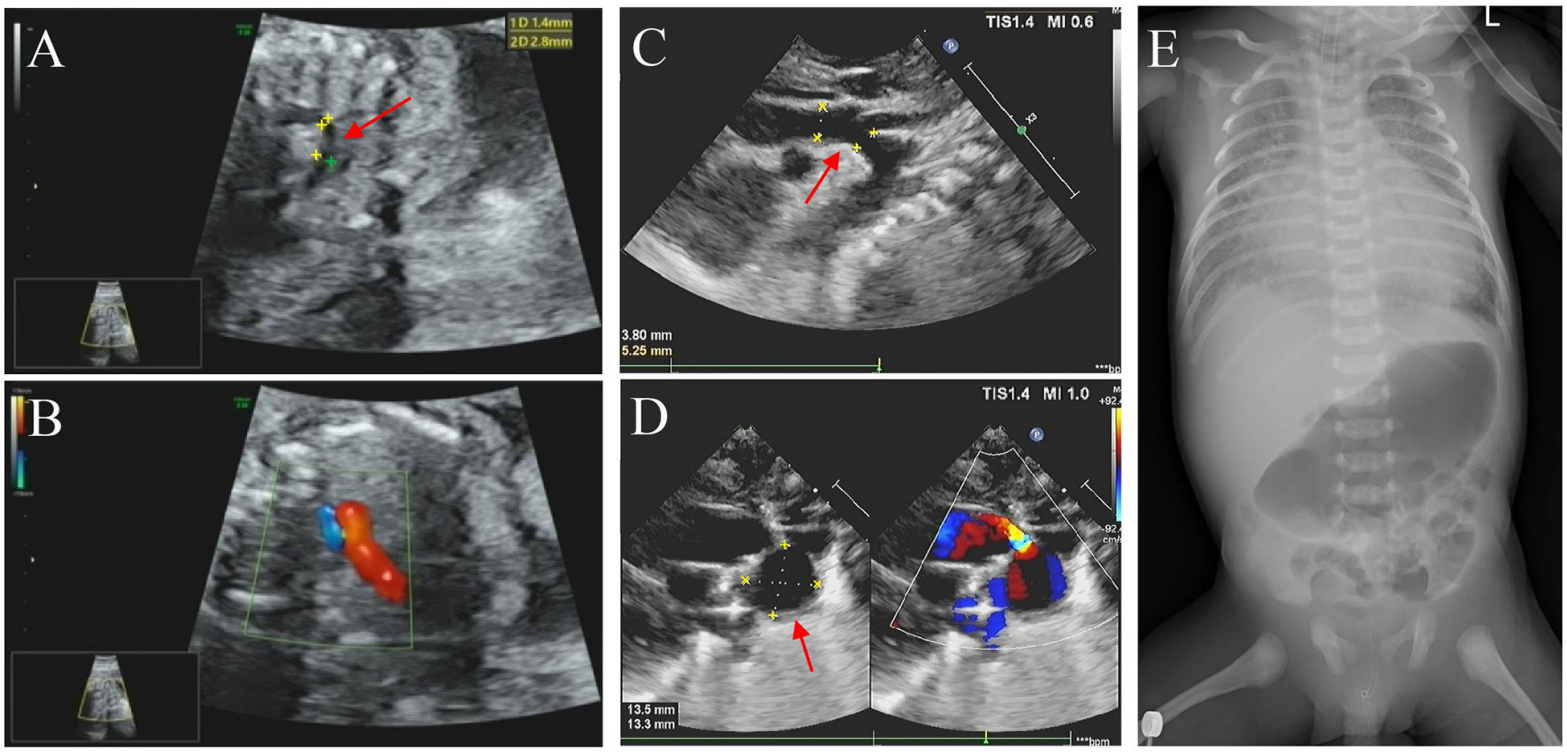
Imaging examination results. (A,C) Echocardiography performed during the fetal period (25 weeks pregnant) and neonatal period, respectively, showed the coarctation of the aortic arch (marked with an arrowhead). (B) The fetus exhibited pulmonary and aortic blood flow incongruity with an increased vessel ratio. (D) Neonatal imaging showing a patent ductus arteriosus aneurysm (marked with an arrowhead) and a bidirectional arterial shunt. (E) Digital x-ray imaging did n't show any obvious developmental abnormalities of the sternum, ribs, or spine.
